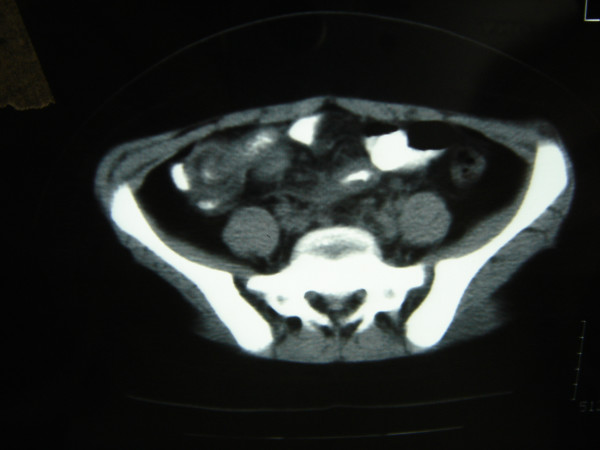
In the CT scan remaining of the contrast liquid in cecum as well as oedema of the cecal wall were observed suggested peri-appendiceal inflammation.
